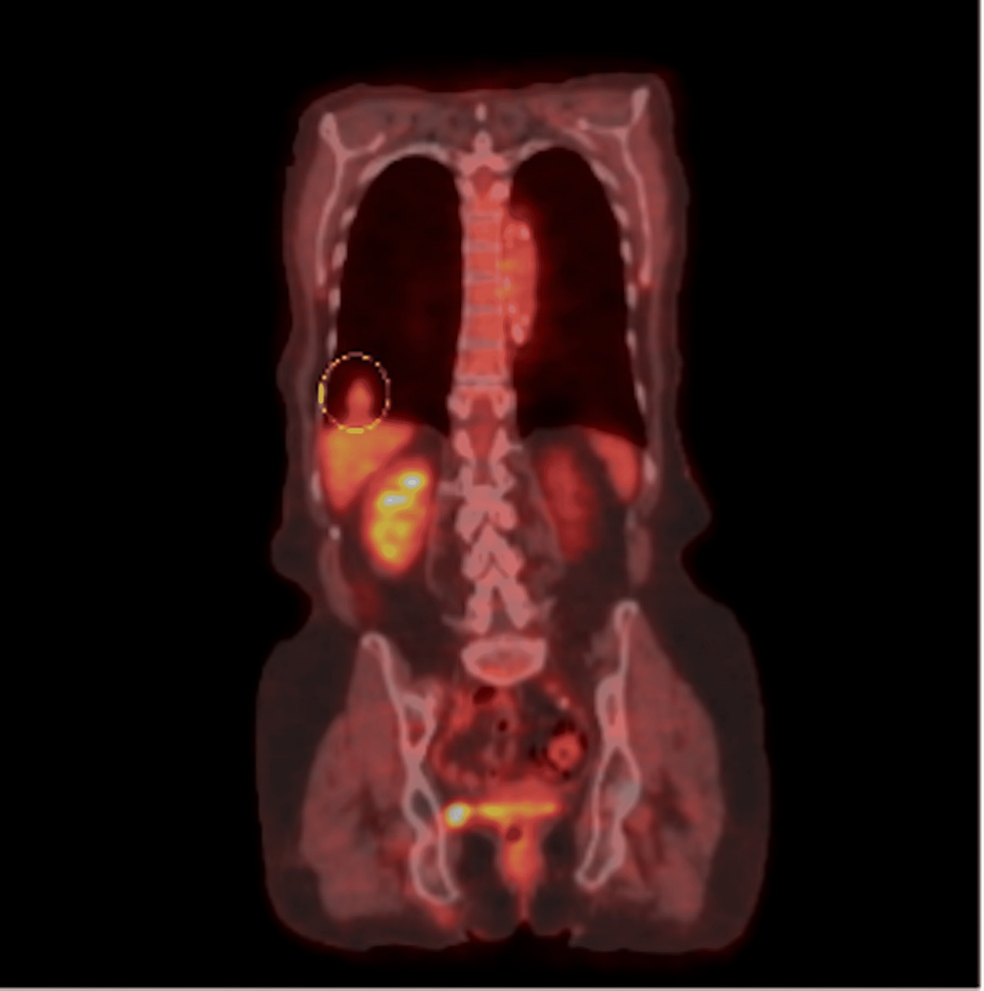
PET scan Hypermetabolic activity within the lesion with a maximum SUV of 2.6 without evidence of nodal involvement
